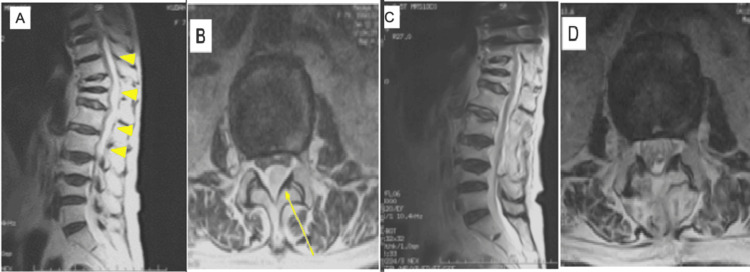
Pre- and postoperative MRI showing resolution of epidural hematoma in Case 1. (A) Preoperative sagittal T2-weighted MRI showing a hyperintense epidural mass extending from T6 to L4 (yellow arrowhead). (B) Preoperative axial T2-weighted MRI showing dorsal epidural hematoma compressing the thecal sac (yellow arrow). (C) Postoperative sagittal T2-weighted MRI demonstrating decompression of the spinal cord. (D) Postoperative axial T2-weighted MRI showing resolution of the hematoma and decompression of the spinal cord MRI: magnetic resonance imaging
